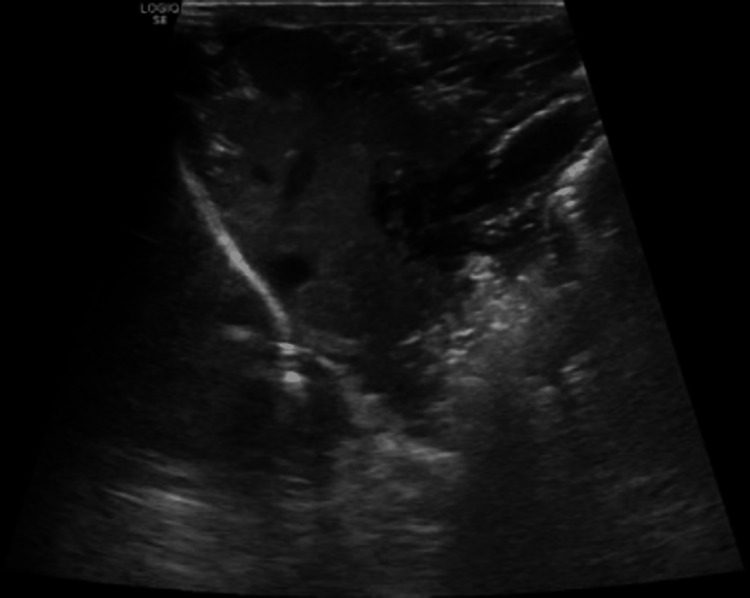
Abdominal ultrasound showing liver is normal in size. The presence of innumerable hypoechoic foci distributed in portal venous tracts and liver parenchyma. Periportal attenuation with nonvisualization of portal vein wall, collapsed IVC and attenuated hepatic vein also evident. Pericholecystic edema along with air in the gallbladder wall.
